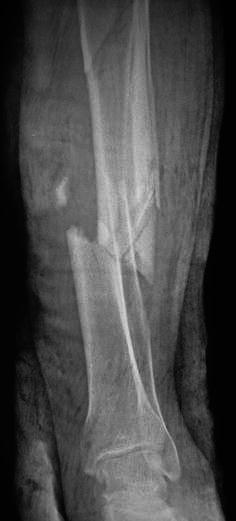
Preoperative radiograph of the fractured left tibia
